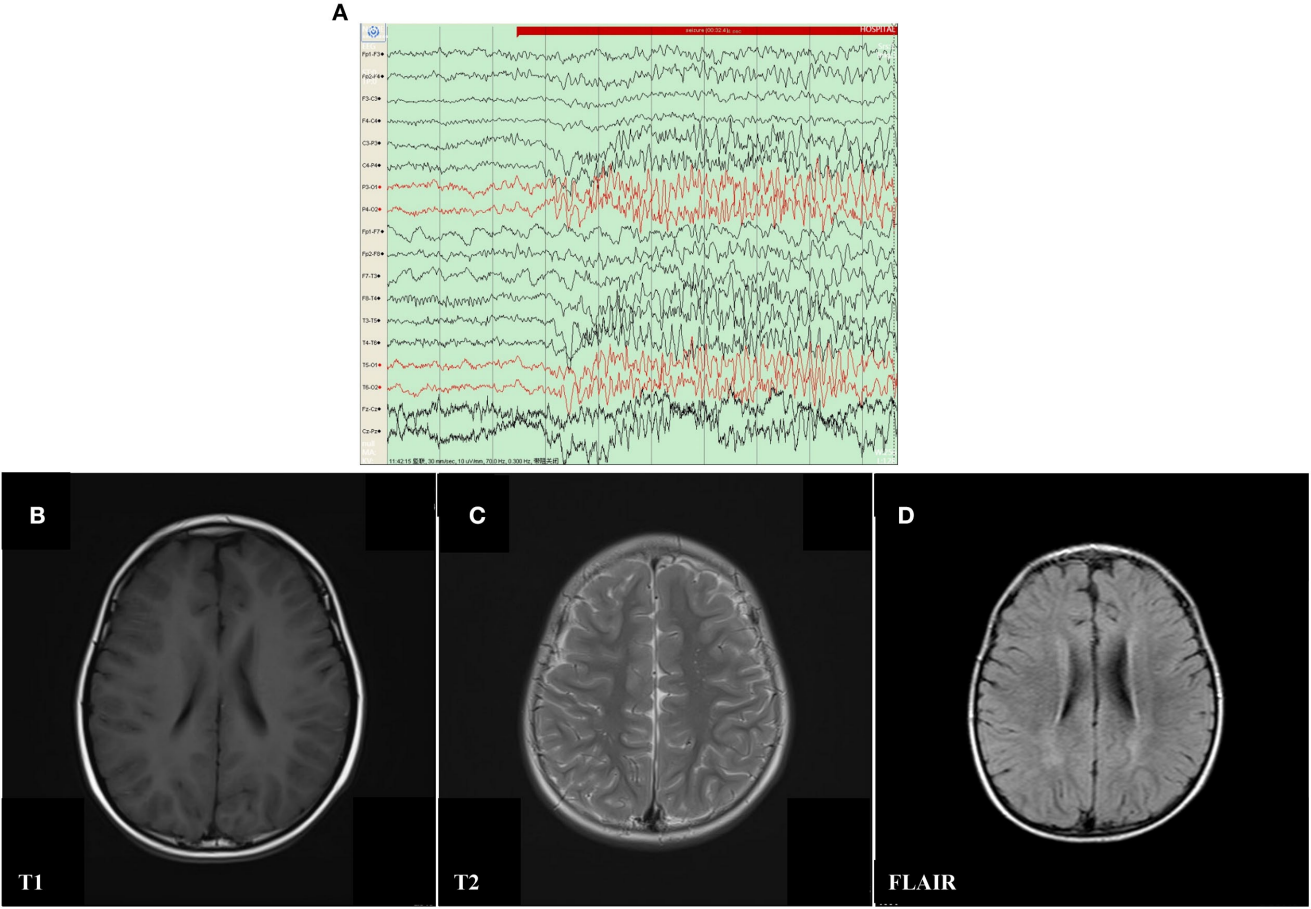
Electroencephalography (EEG) and Cranial magnetic resonance imaging (MRI) result of proband with mosaic mutation in PCDH19 gene (c.840C>A, p. Tyr280*). (A) The analysis of electroencephalography (EEG) exhibited that sharp waves and sharp slow complex waves in the bilateral parietal, occipital, and posterior temporal regions were distributed locally with normal background during the interictal period. (B–D) Punctate white matter lesions appeared with slightly long T1 and T2 signals, as well as slightly high signal on FLAIR in the periventricular white matter and slightly plump bilateral ventricles.
